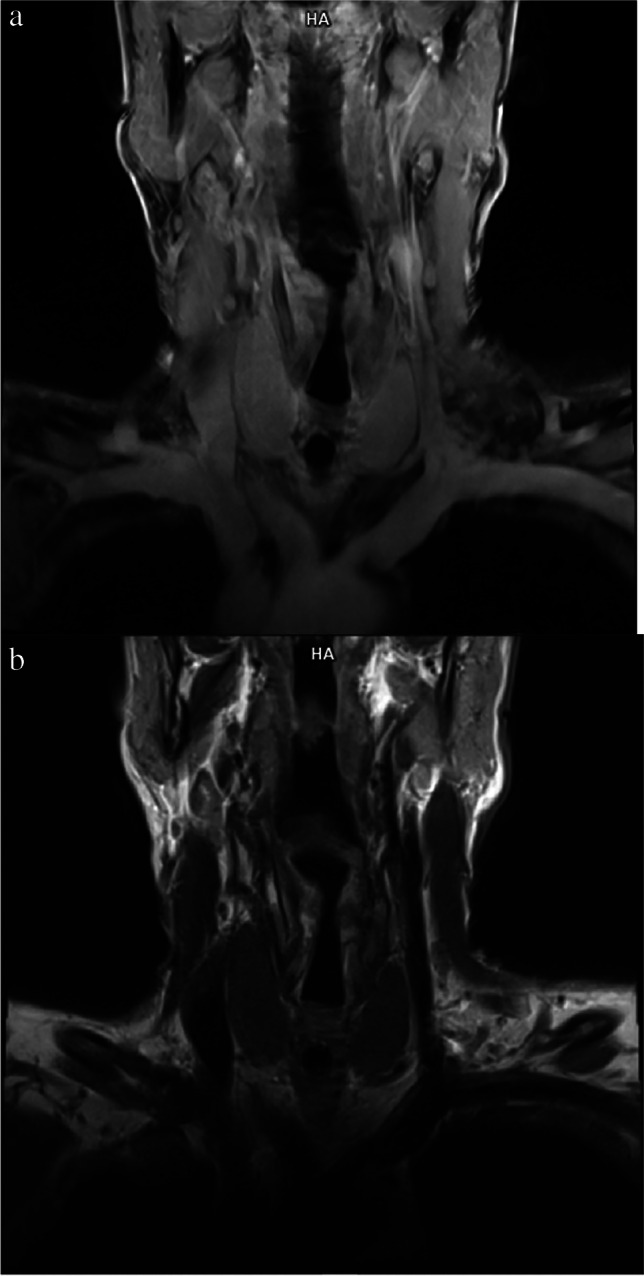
Coronal T1-weighted MRI without (a) and after paramagnetic contrast application (b). Normal thyroid with homogenous slightly hyperintense signal compared to strap muscle was visible before contrast injection and homogenous increase of the signal after injection of the contrast was noted
